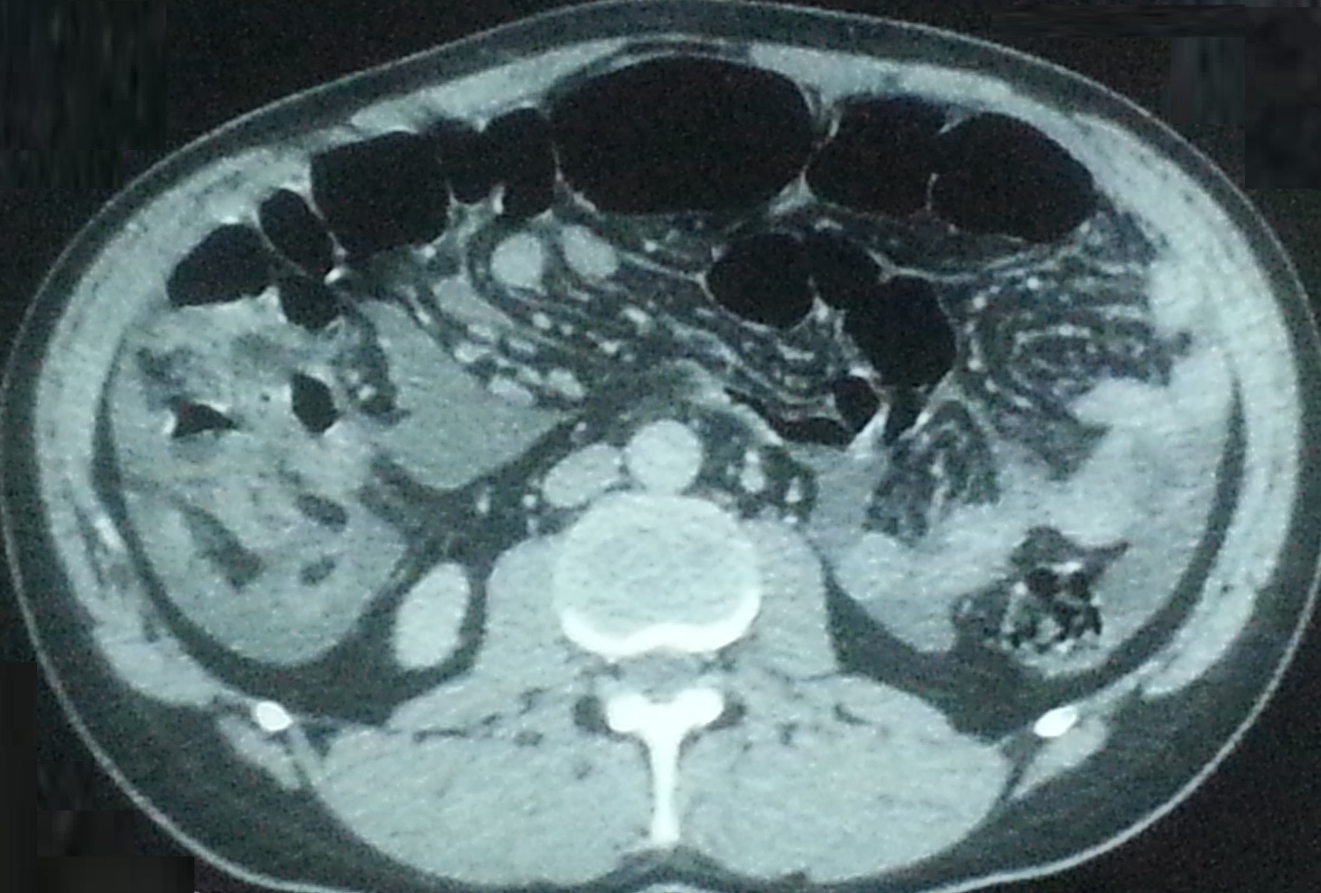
Complete regression of both the ascites and the peritoneal micronodules in computed tomography of the abdomen.
